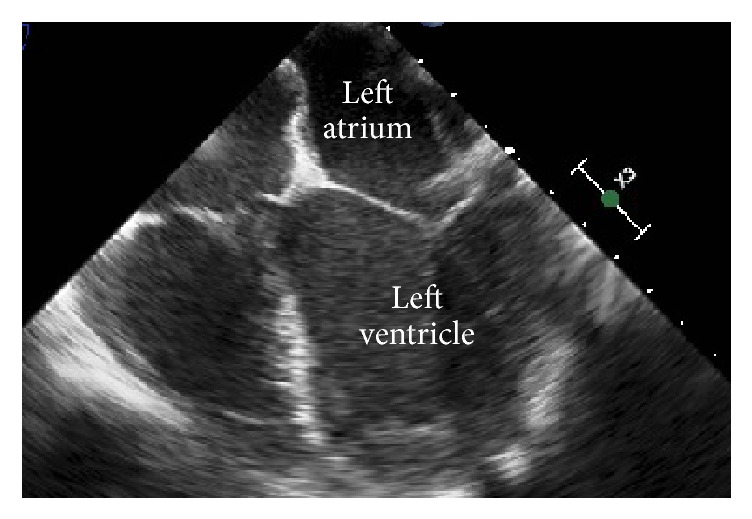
Transesophageal echocardiogram (TEE) image showing a preserved mitral valve after surgery resection of the CPFE.
